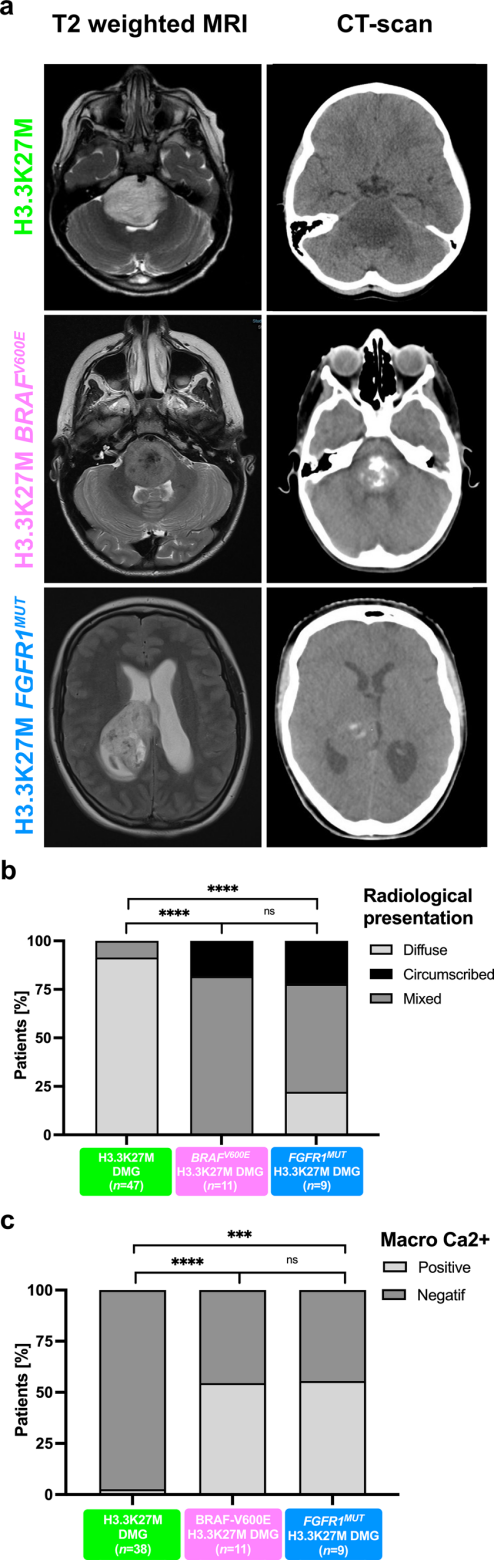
Radiological specificities of DMG H3-K27M *BRAF*^*V600E*^* or FGFR1*^*MUT*^. a T2-FLAIR (Fluid-attenuated inversion recovery) MR images sequences or CT scans (computed tomography) of DMG H3-K27M according to *BRAF* or *FGFR1* mutation status. b Comparison of the tumour radiological presentation (diffuse, circumscribed, or mixed) of DMG according to their genotype (chi-square test for trend: ns, *p* value < 0.0001). c Comparison of presence of macro-calcifications in DMG CT-scans according to the presence of MAPK alteration (Fisher’s exact test: *p* value < 0.0001, p* = 0.0210, p* = 0.0348)
